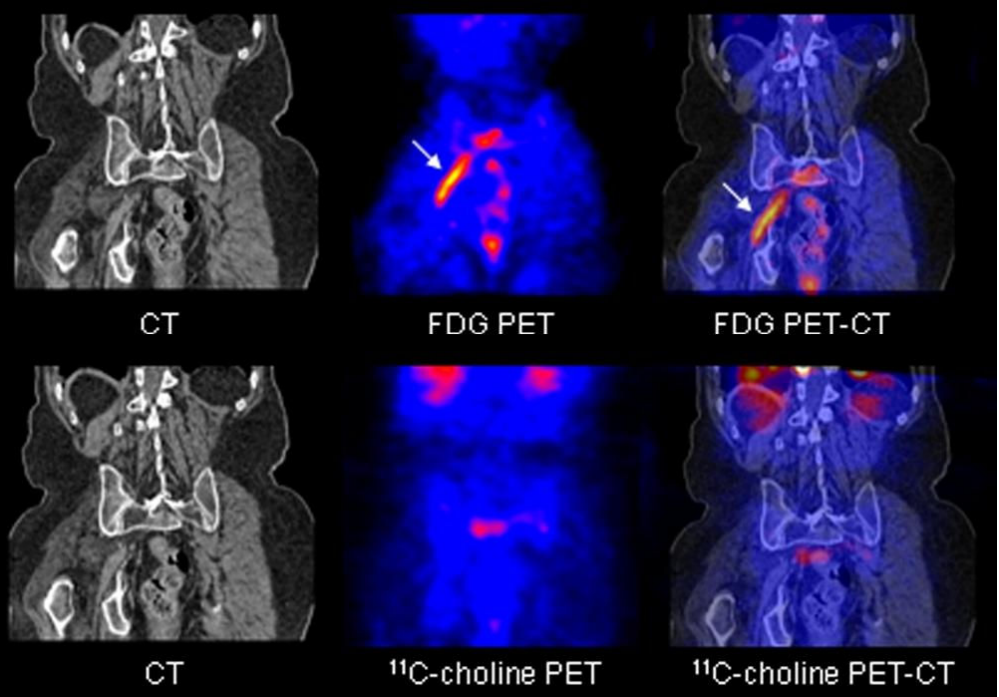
Upper row shows increased FDG uptake in the right sacral plexus (arrow) on the fused FDG PET and fused FDG PET-CT scan. Lower row shows normal ^11^C-choline distribution in the right sacral plexus region.
